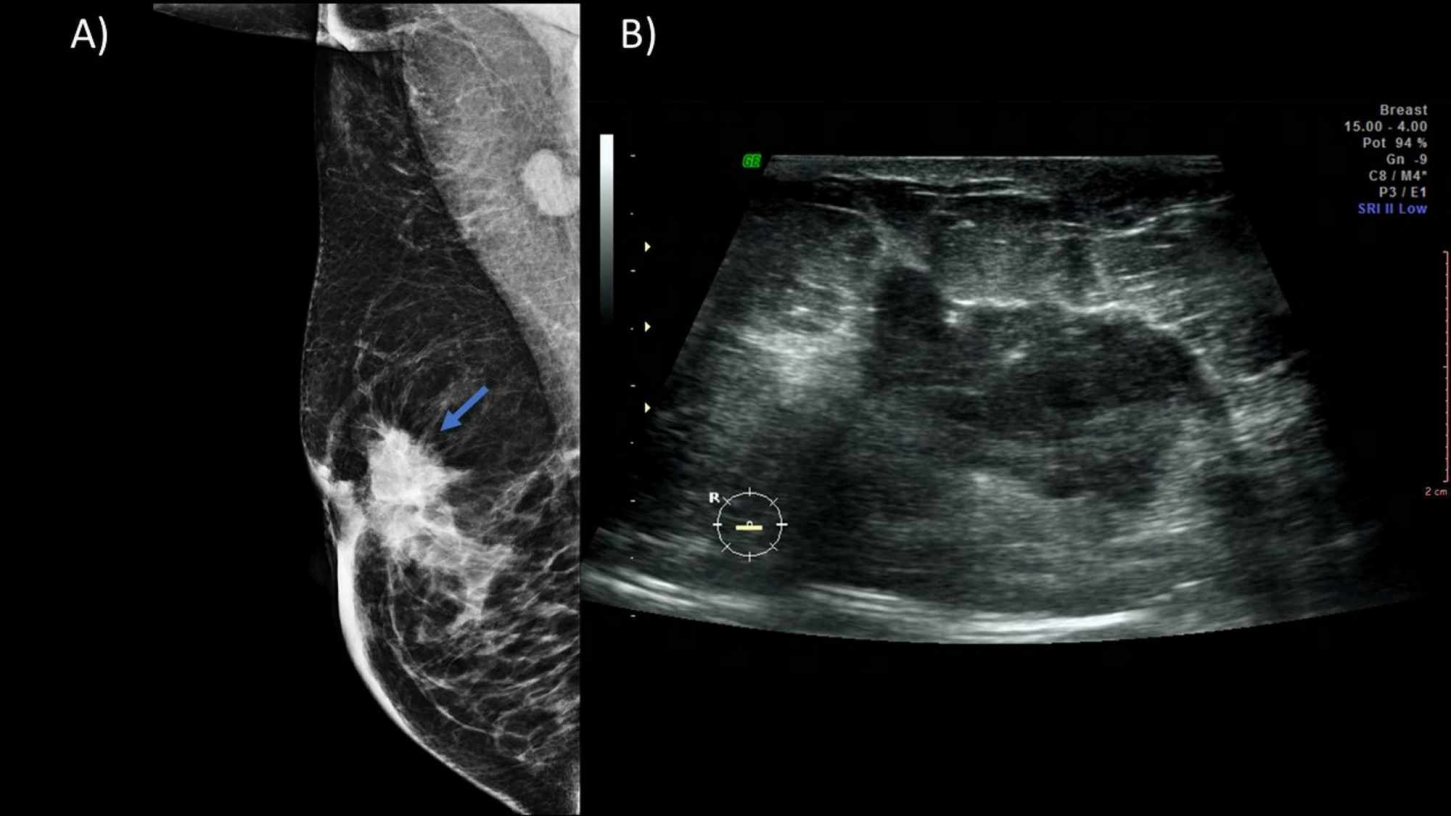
A 35-year-old patient in the immediate puerperium with a palpable nodule in the right breast. (A) MLO projection of the right breast showing a hyperdense nodule (blue arrow) in the retroareolar region of spiculated margins that conditions skin thickening and retraction. (B) Ultrasound showing a hypoechoic nodule of 5 cm, spiculated borders in the retroareolar region. A percutaneous biopsy was performed with a moderately differentiated infiltrating ductal carcinoma result. MLO, mediolateral oblique
